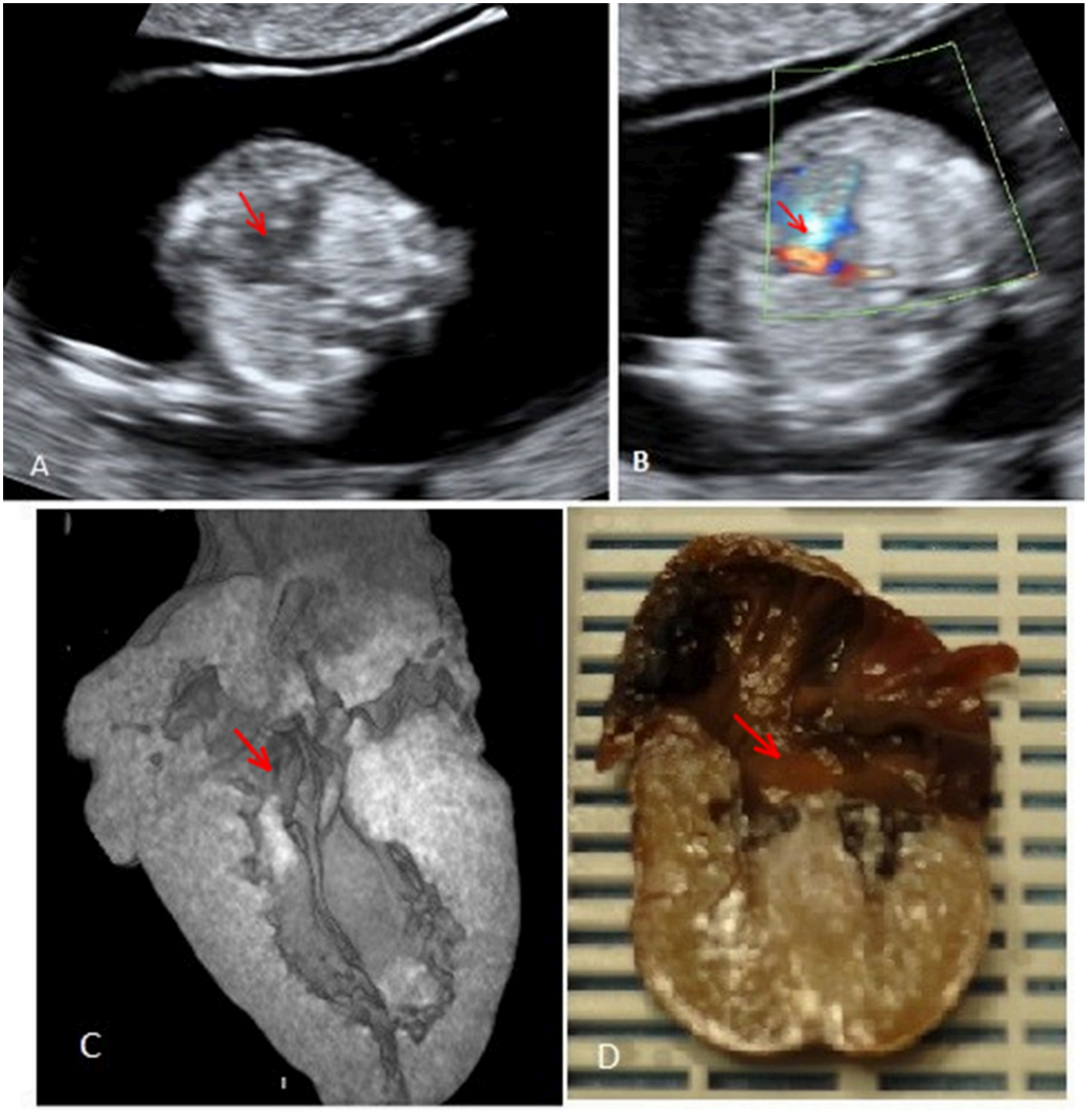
Case 1 (atrioventricular septal defect): (A) Prenatal fetal echocardiography: four chamber view showing the atrioventricular septal defect. (B) Prenatal fetal echocardiography: four chamber view with color Doppler confirmation of the defect. (C) Post-mortem micro-CT: four chamber view showing the atrioventricular septal defect. (D) Conventional autopsy: coronal section showing the four chamber view and the atrioventricular septal defect. Red arrows mark the atrioventricular septal defect.
